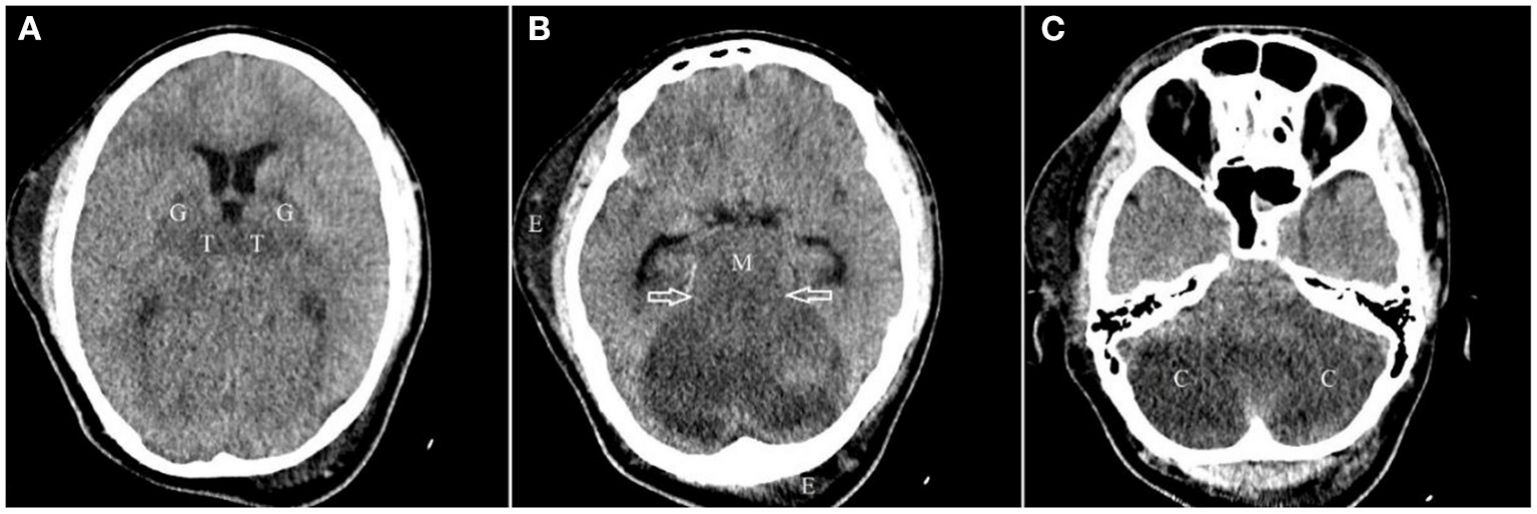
Axial images of non-contrast brain CT scan from top to bottom (A–C) demonstrate near symmetric hypodensity involving both thalami (T) and globi pallidi (G), bilaterally, midbrain (M) and both cerebellar hemispheres (C) with evidence of ascending transtentorial herniation [hallow arrows in (B)]. Subcutaneous edema (E) in right temporal and left occipital regions are also seen.
